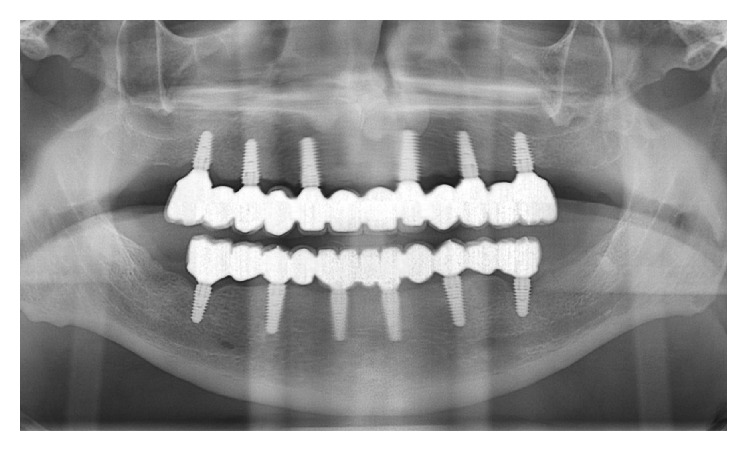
Panoramic radiograph of the FFAs at the 3-year follow-up control.
